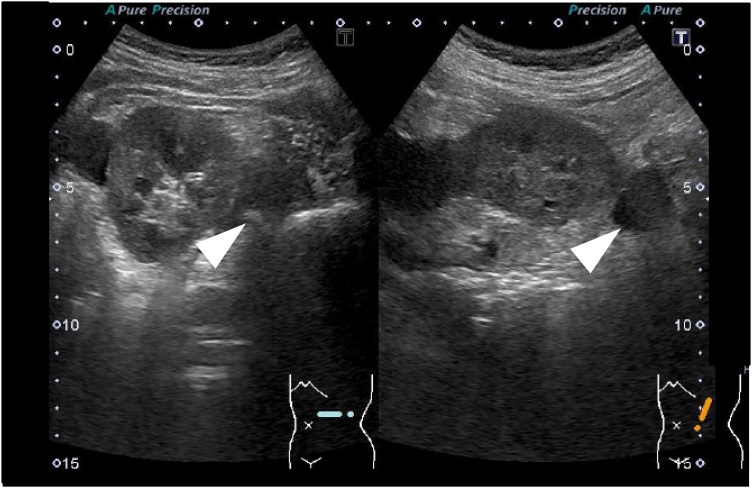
Abdominal ultrasonography shows the presence of a solid mass in close approximation to the kidney.
